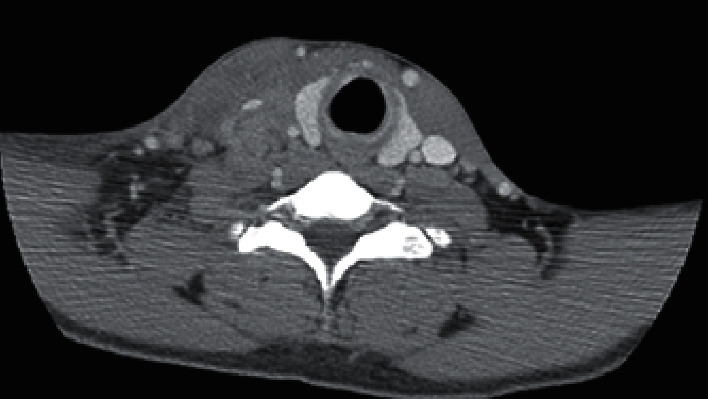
Pretreatment computed tomography of neck soft tissue with contrast, axial image shows right internal jugular vein compressed to a slit by a large heterogenous jugulo-digastric mass.
